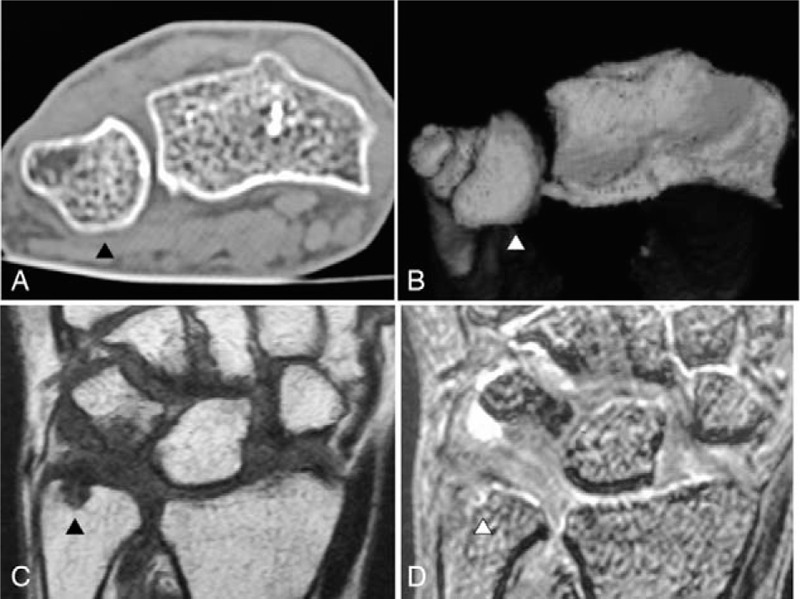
Imaging of the distal forearm. Select coronal cut of computed tomography (A) and three-dimensional computed tomography reconstruction (B) showing subluxation of the ulnar head to the anterior side of the sigmoid notch on the radius (arrowhead). Select coronal cut of magnetic resonance imaging of the wrist (C and D). The void in the ulnar head (arrowhead) indicates the disruption of the ulnar-side triangular fibrocartilage complex (TFCC) on a coronal proton density-weighted image (C). The high intensity region of the TFCC (arrowhead) indicates the avulsion of the ulnar-side TFCC on a coronal gradient recalled echo T2-weighted image (D).
